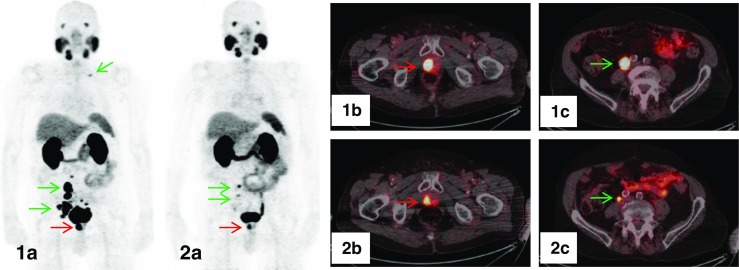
Follow-up ^68^Ga-PSMA-11 PET/CT (low-dose CT) of an 80-year-old CRPC-patient who had received treatment with ^177^Lu-PSMA-617. On the PET scan prior to therapy, local tumor in the prostate bed (*red arrow*), multiple abdominopelvic and one cervical LN metastases (*green arrows*) were clearly visible on maximum intensity projection (MIP) (1a) and on fused axial PET/CT-images (1b, 1c). Restaging PET/CT performed 8 weeks after administration of four cycles of ^177^Lu-PSMA-617 with a total accumulated activity of 24.9 GBq showed a significant reduction of the primary tumor (*red arrow*) and an impressive partial response of LN metastases with only small metastases left in the right iliac region (*green arrows*), as displayed on MIP (2a) and fused axial PET/CT-images (2b, 2c)
